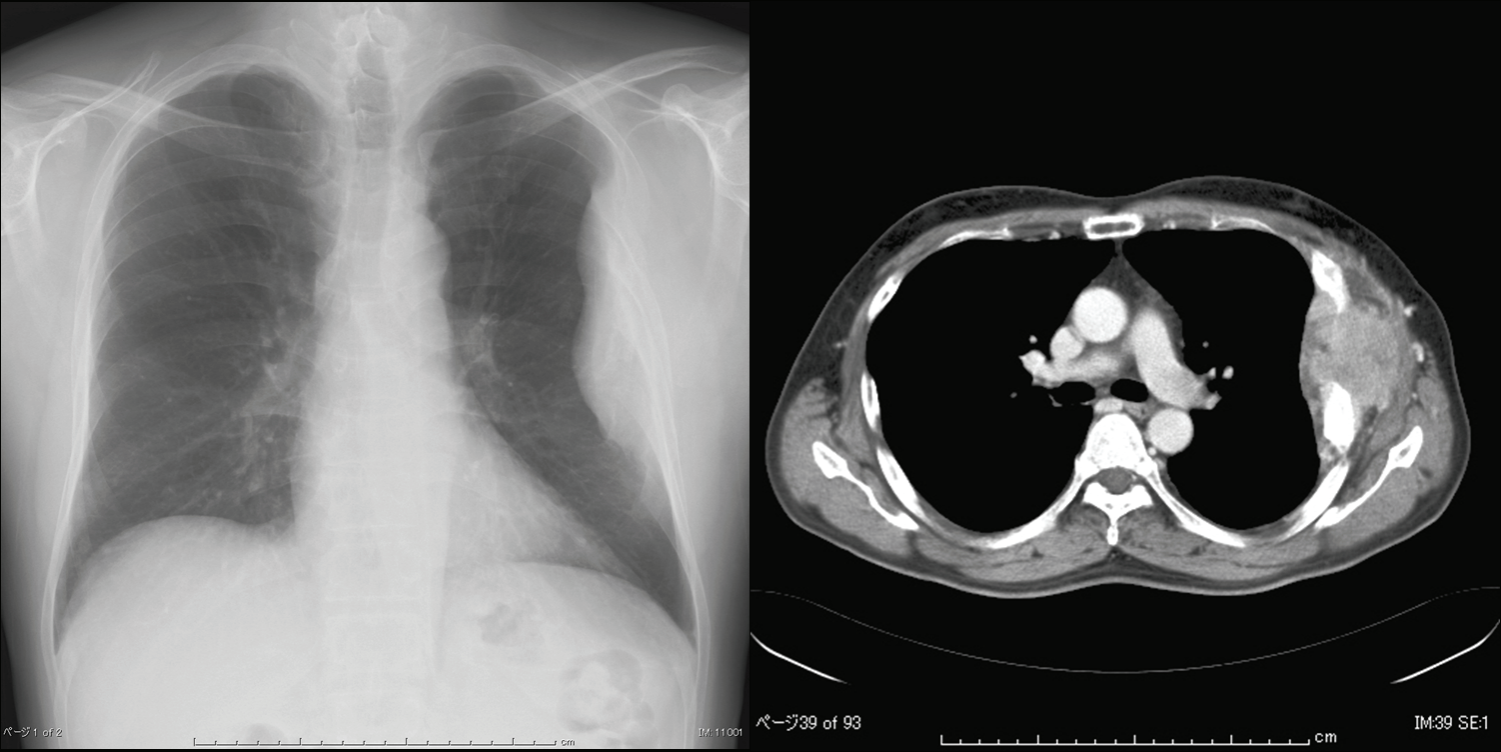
Chest X-ray and computed tomography scan before surgery. Chest X-ray and computed tomography scan showed a 14 × 8 cm tumor of the left chest wall in the left ribs.
